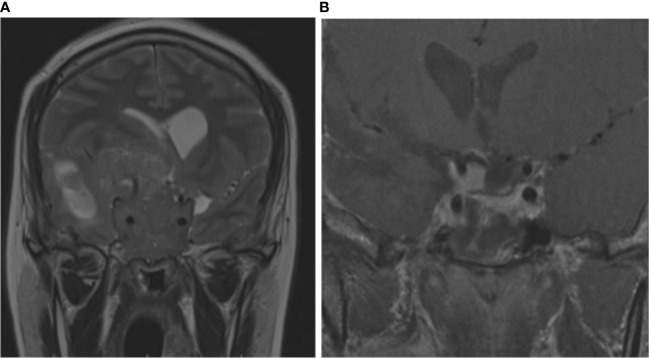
Coronal and sagittal MRI of individual III.2 (family 4). Giant pituitary adenoma with suprasellar extension reaching the right frontoparietal region, with invasion of cavernous sinus and third ventricle (A). Six months after surgery and cabergoline, the tumor exhibited significant shrinkage and necrosis (B).
